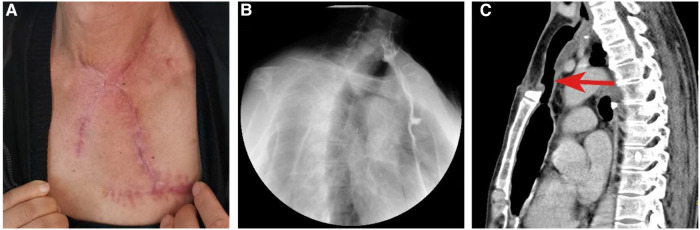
Postoperative visual inspection, esophagography and CT scan. (A) Postoperative visual inspection of external neck skin. (B) Esophagography shows the function of the reconstructed esophagus at the second month postoperatively. (C) CT shows the spacious reconstructed esophagus at the second month postoperatively.
